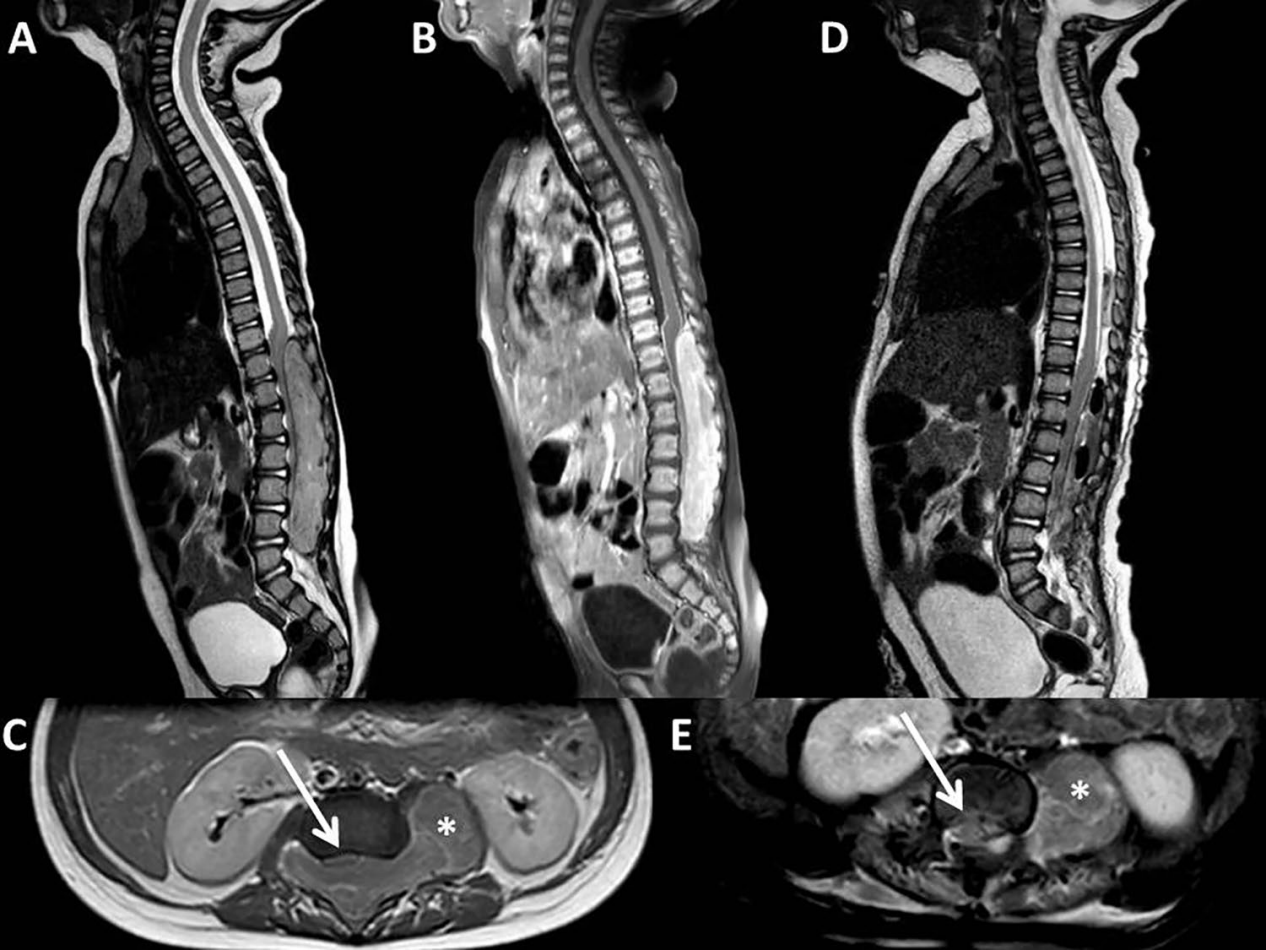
Preoperative sagittal T2-weighted and T1-weighted after gadolinium MR (A and B, respectively) showing thoracolumbar intracanal neuroblastoma. Preoperative axial T1-weighted after gadolinium MR (C) highlighting the anterior displacement and severe compression of the dural sac (*arrow) *by the intracanal component of the tumor and the extraforaminal component of the tumor extending through the left neural foramen (*asterisk*). Postoperative sagittal T2-weighted and axial T1-weighted after gadolinium MR (D and E, respectively) confirming the complete resection of the intracanal tumor, with expansion of the dural sac (*arrow*), with the extraforaminal component of the tumor still in place (*asterisk*)
